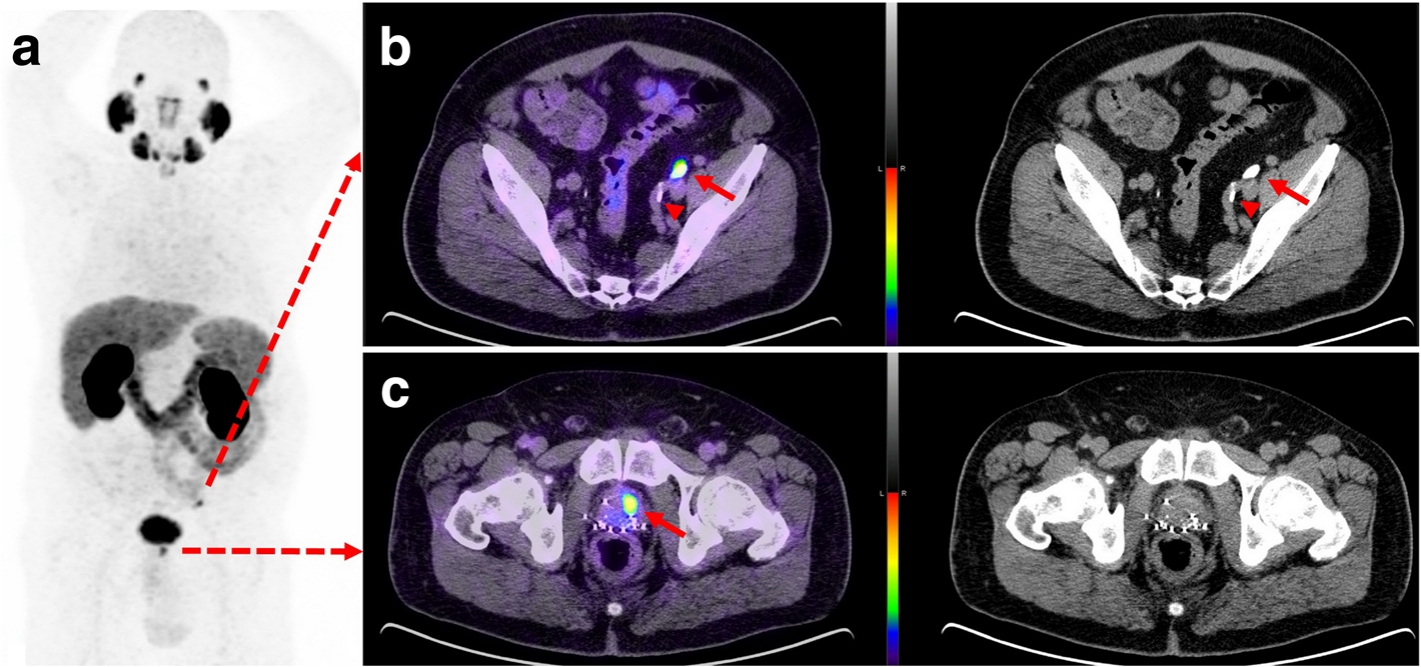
Sixty-six-year-old man with BCR and PSA 3.2 ng/L following brachytherapy. Panel a. PSMA PET MIP image demonstrates two foci of activity. Panel b. PET/CT-U and CT-U axial images show focal activity in the left pelvis corresponding to opacified, ectatic ureter (arrows). High density focus more posteriorly relates to a calcified plaque in the iliac vessel (arrow head). Panel c. PET/CT-U and CT-U axial images show focal uptake in the left side of prostate, consistent with local recurrence
